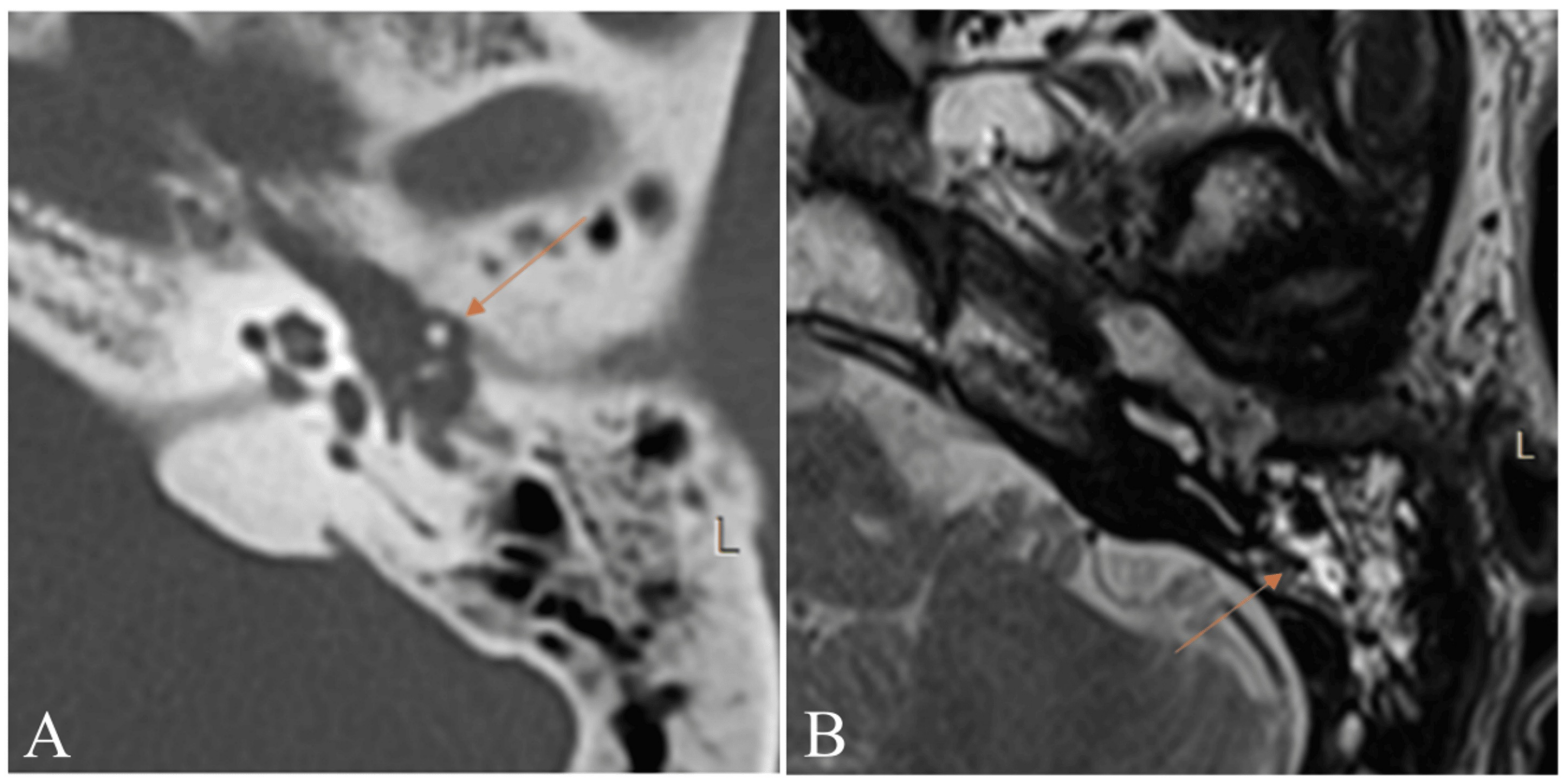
Imaging of case 1. (A) High-resolution axial CT of the internal auditory canal (IAC) without contrast showing a tumor surrounding the left ossicular chain. (B) T2-weighted MRI showing a tumor in the left middle ear cavity.
